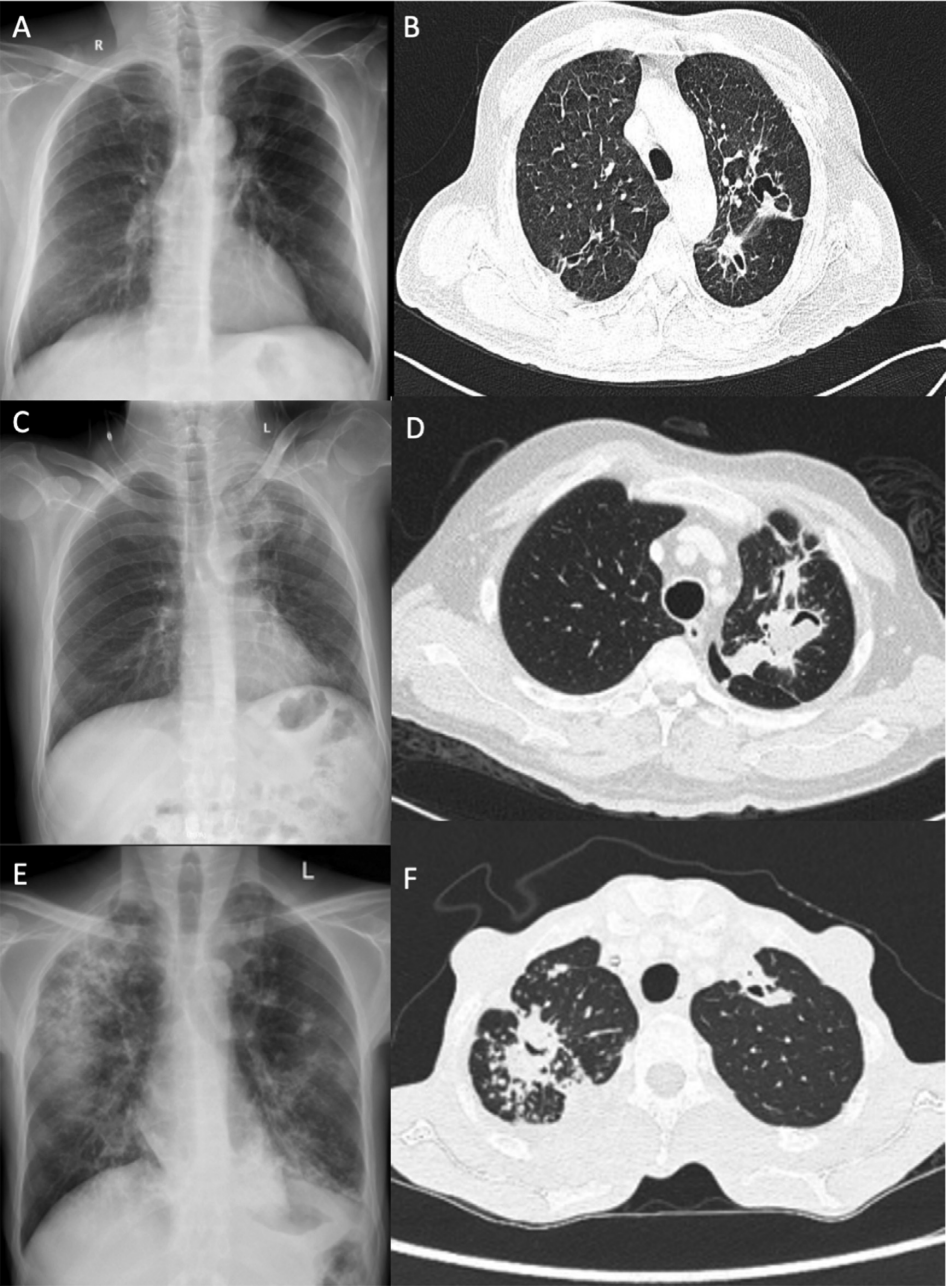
Case 1: 50-year-old male with symptoms of chronic productive cough. (A) Chest radiograph showed reticular opacities in the right upper zone of the lung. (B) Chest CT showed intrabronchial aspergilloma in the left upper lobe. Subsequent tests for IgG against Aspergillus were positive and a diagnosis of CPA was made. Diagnosis of CPA hinges on combined clinico-radio-microbiological features. Case 2: 45-year-old male with past history of tuberculosis presenting with complaints of intermittent hemoptysis. (C) Chest radiograph showed heterogenous opacities in the left upper zone. (D) Chest CT showed air crescent cavity signs in the left upper lobe, suggestive of aspergilloma. Chest radiograph features may be non-specific and advanced chest imaging may be required. Case 3: 30-year-old male with fever and weight loss over a 4-month period. (E) Chest radiograph showed bilateral reticulonodular opacities in the lung, along with consolidation in the right upper and middle zone. (F) Chest CT showed right and left upper lobe cavities, with pleural thickening. Workup for CPA was positive. Presence of pleural thickening is an important marker of the presence of CPA.
